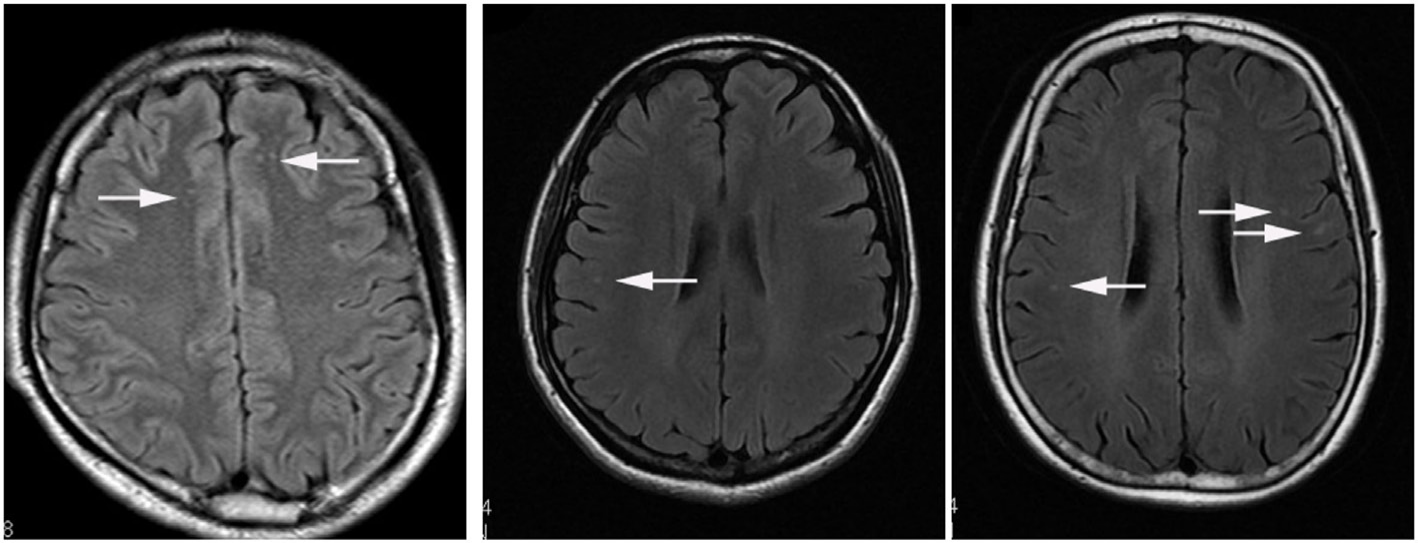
The axial view of typical presentations of different distributions of juxtacortical small lesion/s (JCSL/s) on T2 FLAIR. (A) JCSLs in juxtacortical region of frontal lobe (arrowhead); (B) JCSL in juxtacortical region of right parietal lobe (arrowhead); (C) JCSLs in subcortical region of frontal parietal lobe (arrowhead).
